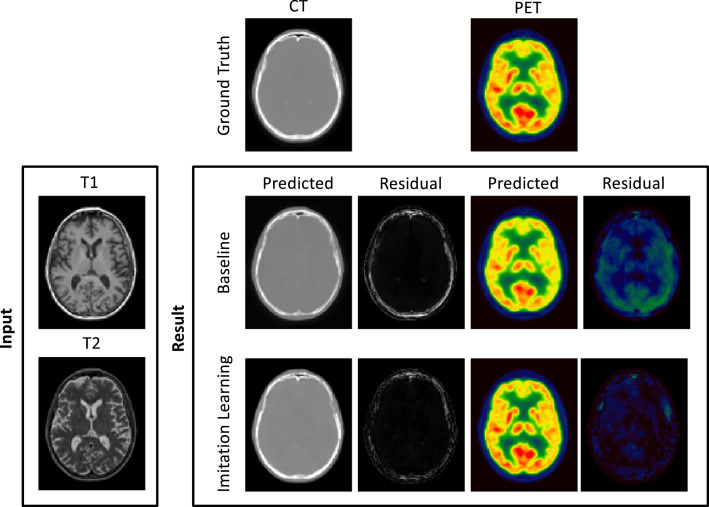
Qualitative results. From top to bottom: Ground-truth, baseline (HighRes3DNet) and imitation learning alongside input MR images. From left to right: CT, pCT-CT residuals, PET, pPET-PET residuals. The error in the pCT generated with the proposed imitation learning is lower than the baseline pCT residuals. The error in the pPET reconstructed with the proposed method is significantly lower than the pPET error for the baseline method.
